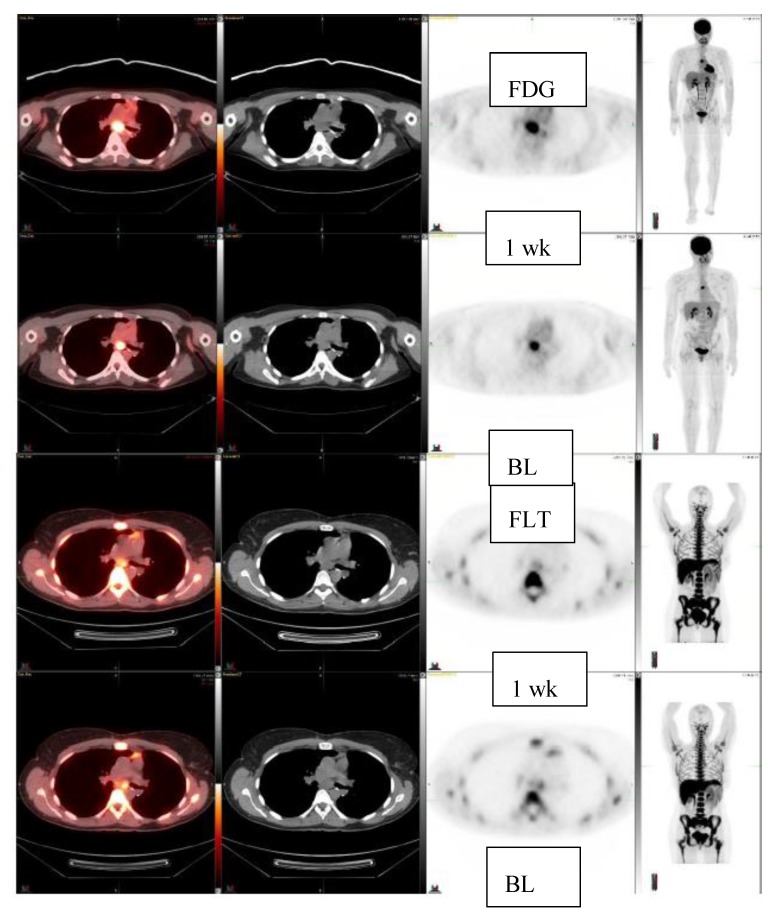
Clear cell sarcoma, left foot, lung resection. FDG-study at baseline and at 1 week (2 upper rows). FLT-study at baseline and at 1 week (2 lower rows). FDG-uptake increases 37% in a subcarinal lymph node: SUVmax 8.9 ->12.2, whereas FLT-uptake decreases 13%, FLT: SUVmax 3.0 ->2.6. On CT it becomes slightly bigger, CT: 2.2 cm × 1.2 cm ->2.3cm × 1.5 cm.
